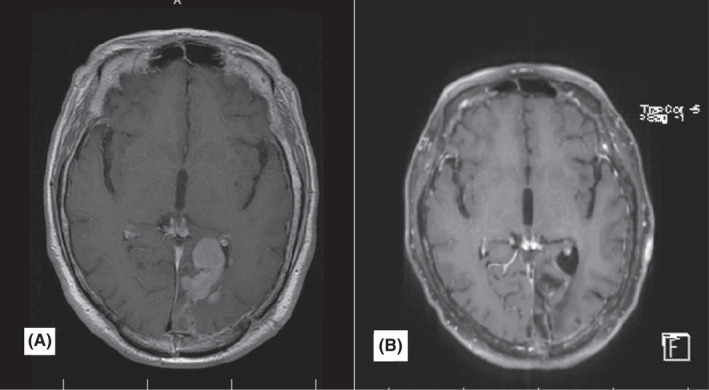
Case number 4. Post‐contrast axial brain MRI (A) brain MRI before treatment. Large mass in the left parietal lobe with avid non‐homogenous contrast enhancement. (B) after a follow‐up of 32 months. Gliomalacia in the left parietal lobe
